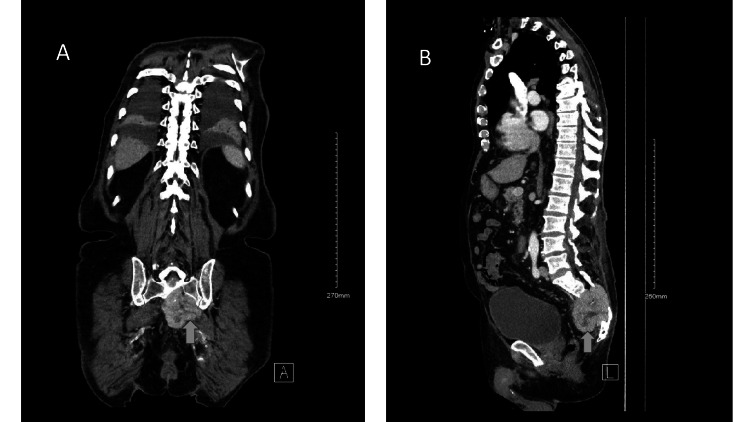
CT abdomen and pelvis with (A) coronal and (b) sagittal images reveal a lobulated sacral mass seen at the sacral vertebrae.
